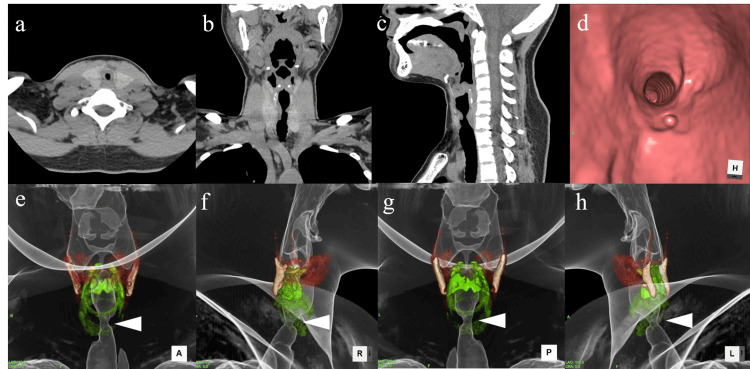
CT findings (a) Axial image. (b) Coronal image. (c) Sagittal image. (d) Virtual endoscopic image. 3D reconstruction images: (e) Anterior view. (f) Right-side view. (g) Posterior view. (h) Left-side view. The subglottic area was narrowed.
